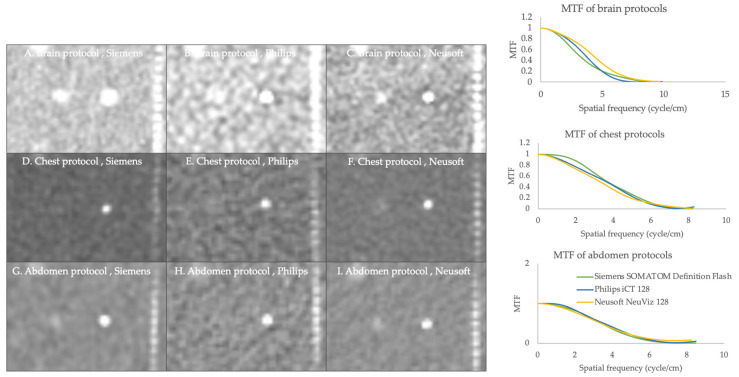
A comparison of the modulation transfer function of brain, chest, and abdomen protocols with various CT scanners. The small wire (indicated by the white dot in the axial image) is used to calculate MTF. The green, blue, and yellow lines represent the Siemens SOMATOM Definition Flash, Philips iCT128, and Nuesoft NeuViz 128, respectively.
